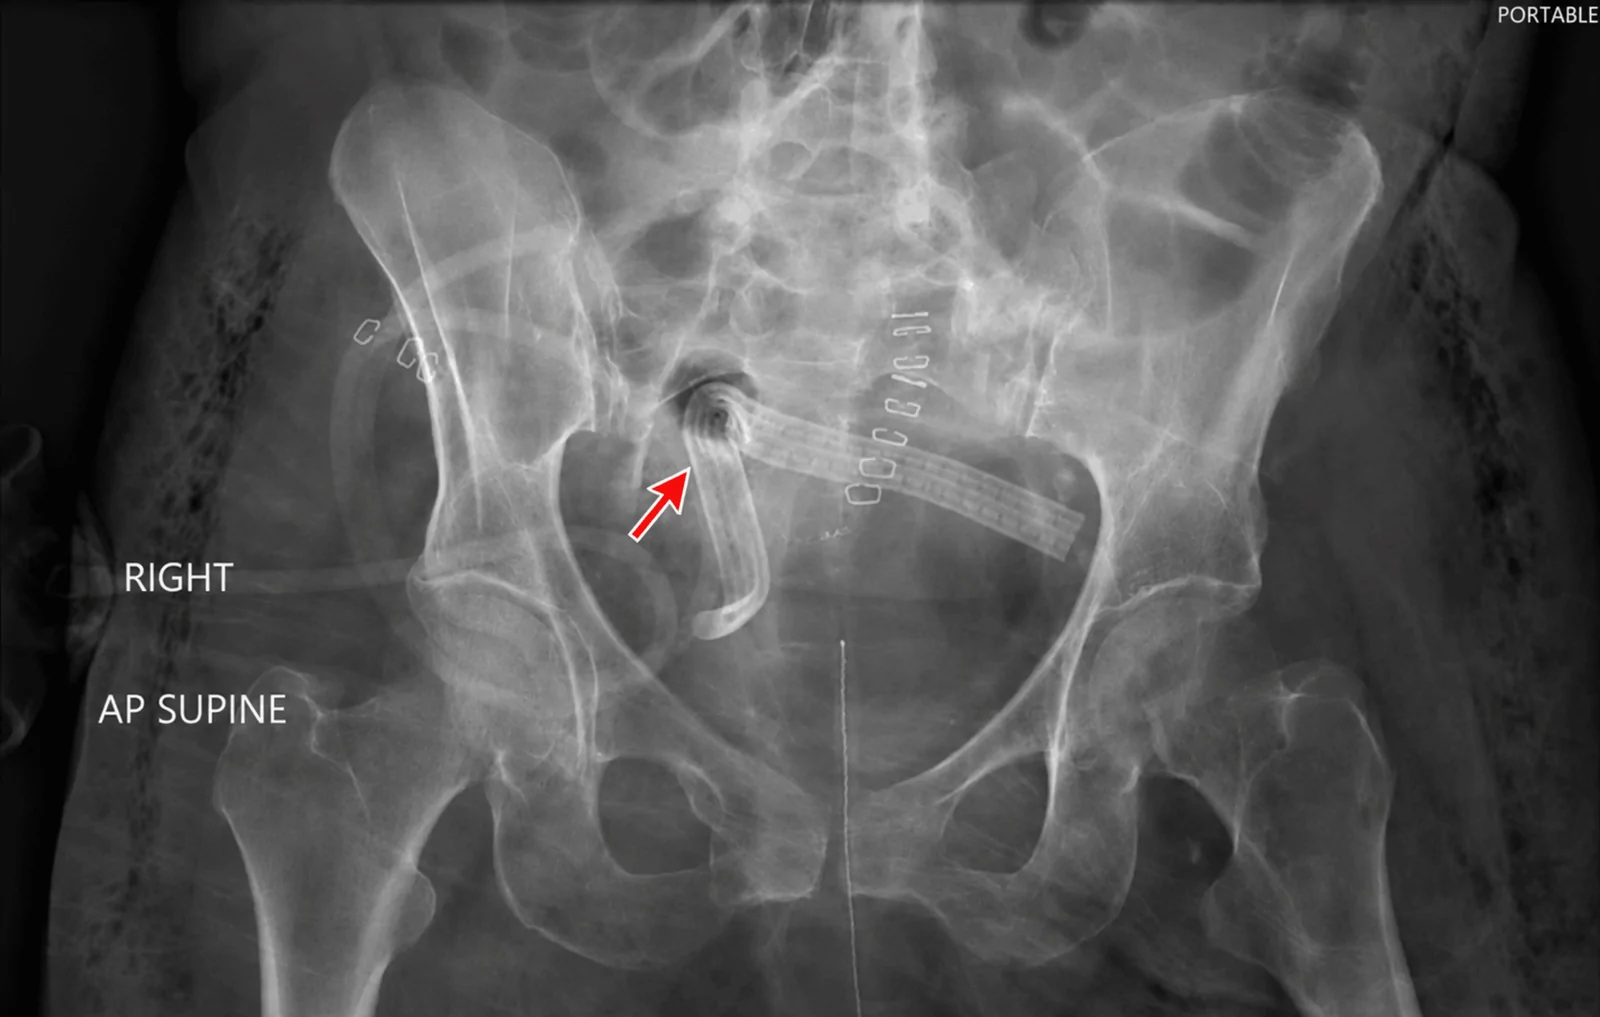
Portable abdominal radiograph The image demonstrates postoperative pelvic drain placement (red arrow) following robotic-assisted low anterior resection and fistula takedown two months after the onset of symptoms
